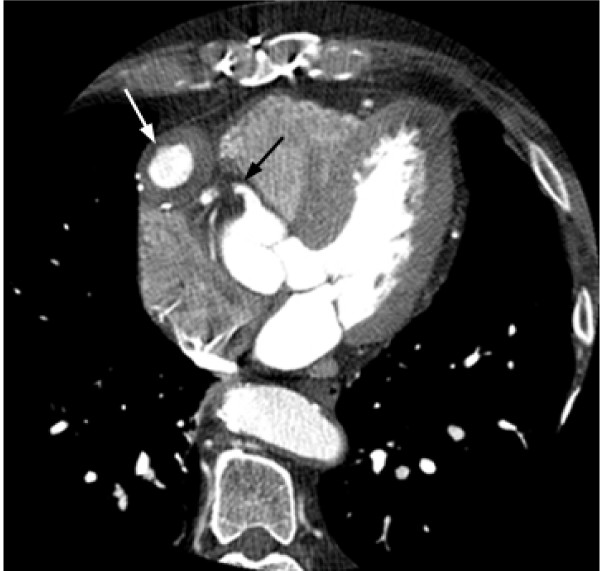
Axial image from CTA demonstrating a 3.9 cm aneurysm of the proximal aspect of the saphenous vein graft in the right AV groove (white arrow). Contrast opacification is seen in the lumen with mass effect on the adjacent native RCA (black arrow).
